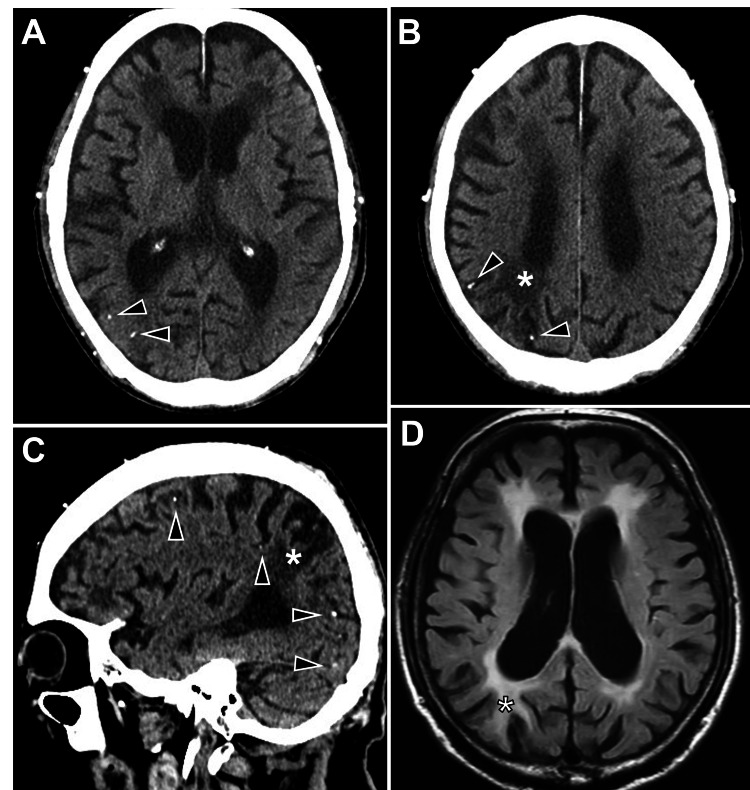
Non-contrast brain CT scans and MRI at the representation Non-contrast brain CT scans demonstrate a low-attenuation region (asterisk) indicative of a chronic infarction in the parieto-posterior watershed area and multiple punctate calcific spots (arrowheads) in the right hemisphere located on the pial surface, exhibiting the characteristic "salted pretzel sign." (A-C) Axial slices. (C) Sagittal slice. (D) Brain MRI shows a chronic infarction in the parieto-posterior watershed area (asterisk). CT: computed tomography: MRI: magnetic resonance imaging
